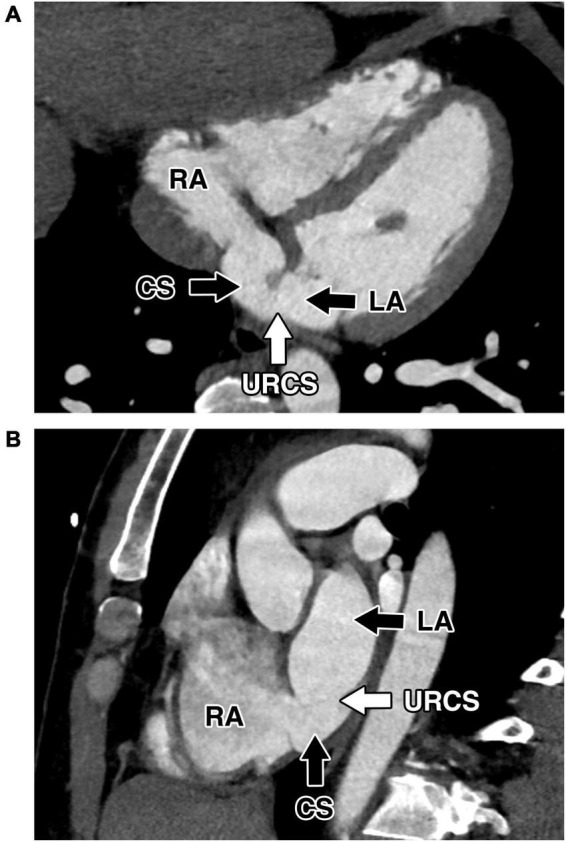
Preoperative cardiac computed tomography. Computed tomography showed extensive communication between the coronary sinus and left atrium caused by partial absence of the roof of the coronary sinus. The absence of the coronary sinus roof and communication between CS and the left atrium. (A) Modified four-chamber projection. (B) Modified short-axis projection. CS, coronary sinus; LA, left atrium; RA, right atrium; URCS, unroofed coronary sinus.
